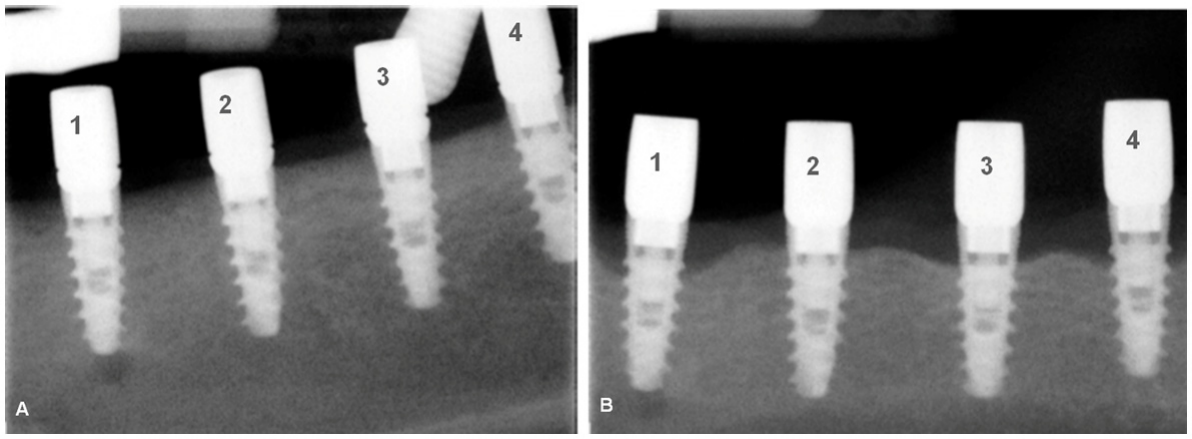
Digital radiographs of implants at the beginning of the plaque formation period in week 24 (A) and at the end of the study in week 46 (B). Differences in crestal bone level are evident. 1. Uncoated zirconia abutment, 2. G3 glassy coated abutment, 3. ZnO35 coated abutment, 4. G1n-Ag coated abutment.
